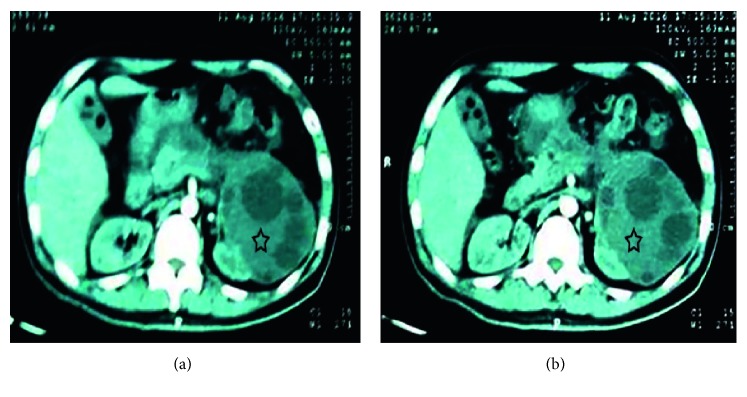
Computed tomography revealing a cystic lesion extending between the body of the pancreas and left kidney (black stars).
